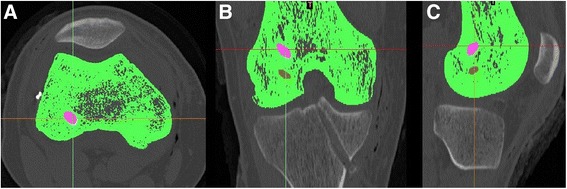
Segmentation process of 2D CT images. Segmentation process performed on the original 2D CT images viewed in axial (A), coronal (B) and sagittal (C) CT images.
